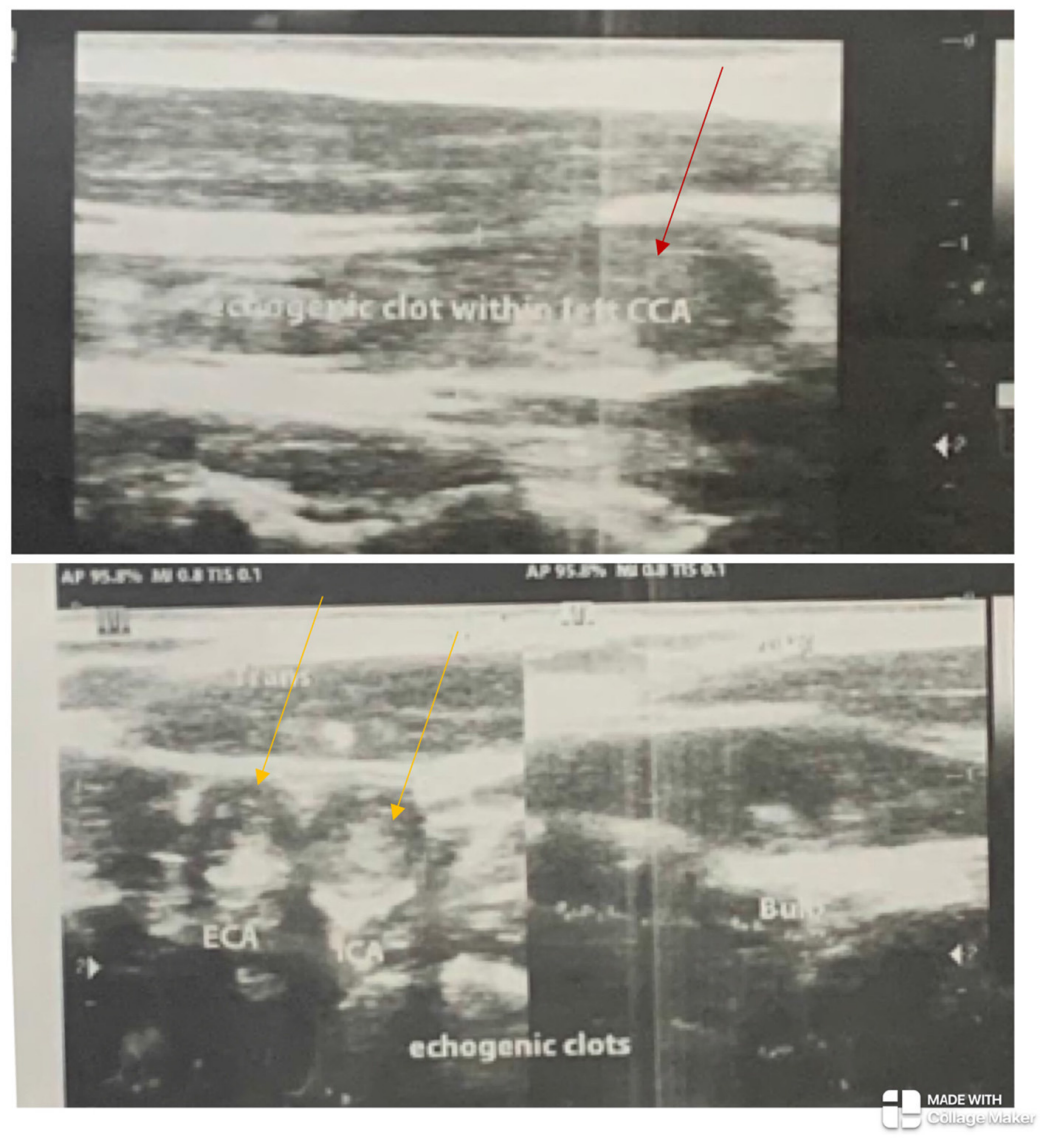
The left common carotid artery (red arrow) and the left internal and external carotid arteries (yellow arrows) show echogenic clots completely occluding their lumina with absent flow on Doppler ultrasonography.
